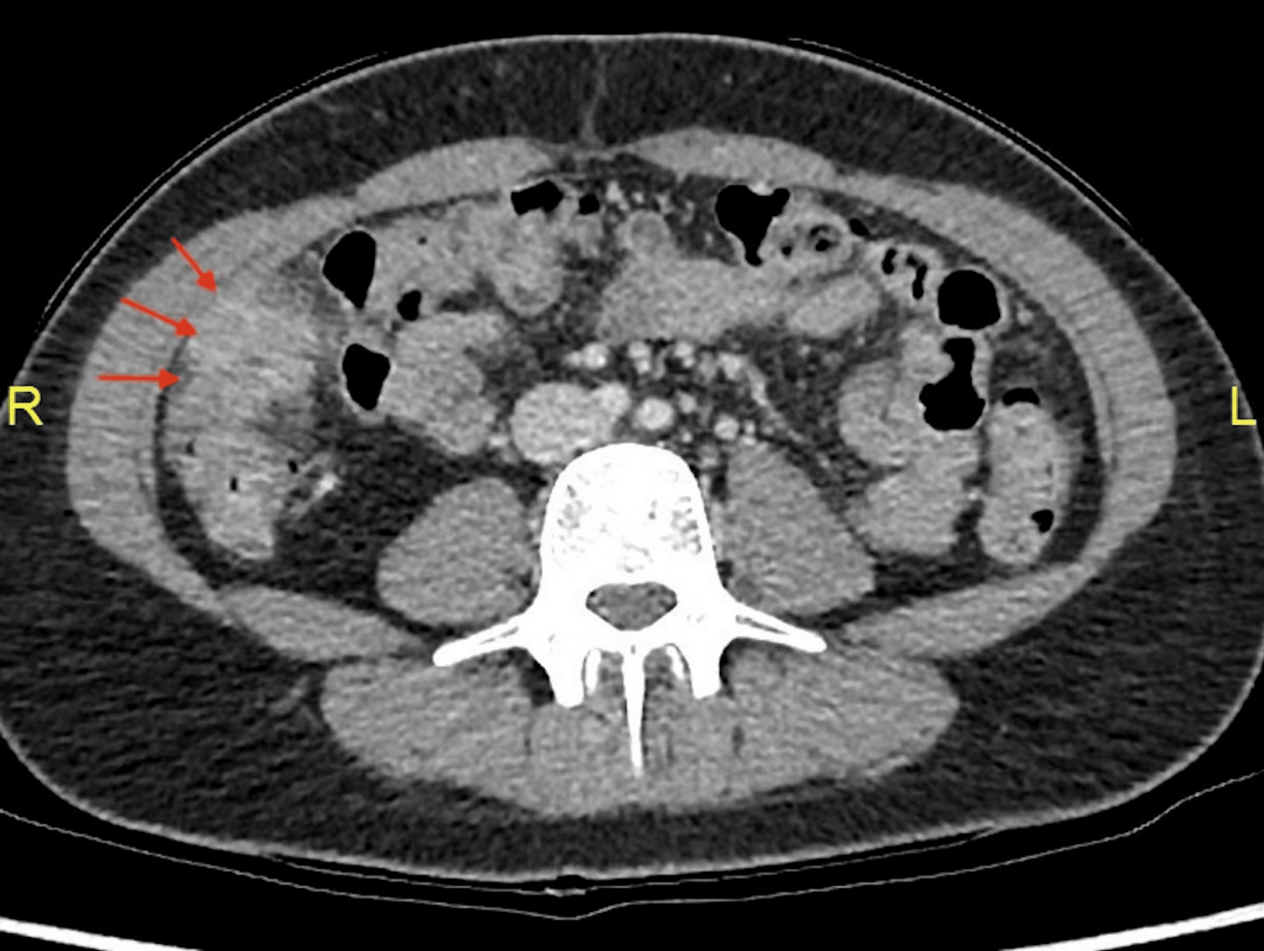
Axial image of the CT abdomen showing an infiltrative inflammatory mass adjacent to the ascending colon.
